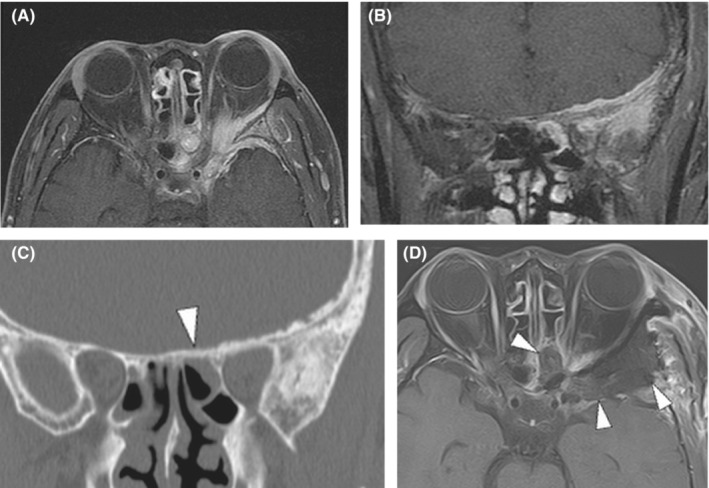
(A, B) Preoperative MRI demonstrating en plaque tumor along the greater wing of the left sphenoid bone and periorbita, extending into the temporal muscle and sphenoid sinus, leading to left exophthalmos. (C) Preoperative CT demonstrating a hyperostosis at the planum sphenoidale (arrowhead). (D) Postoperative MRI demonstrating symmetrical position of eyeball and galeal flap covering from left middle skull base to center anterior skull base (arrowheads). MRI, Magnetic resonance imaging; CI, Computed tomography.
